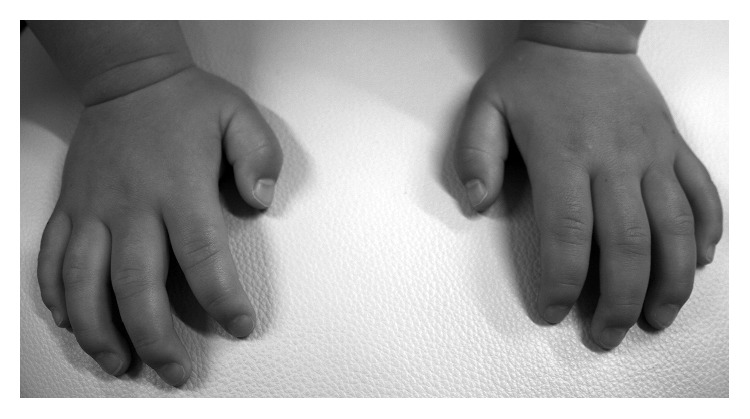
Hand X-ray imaging the clawhand deformity.
